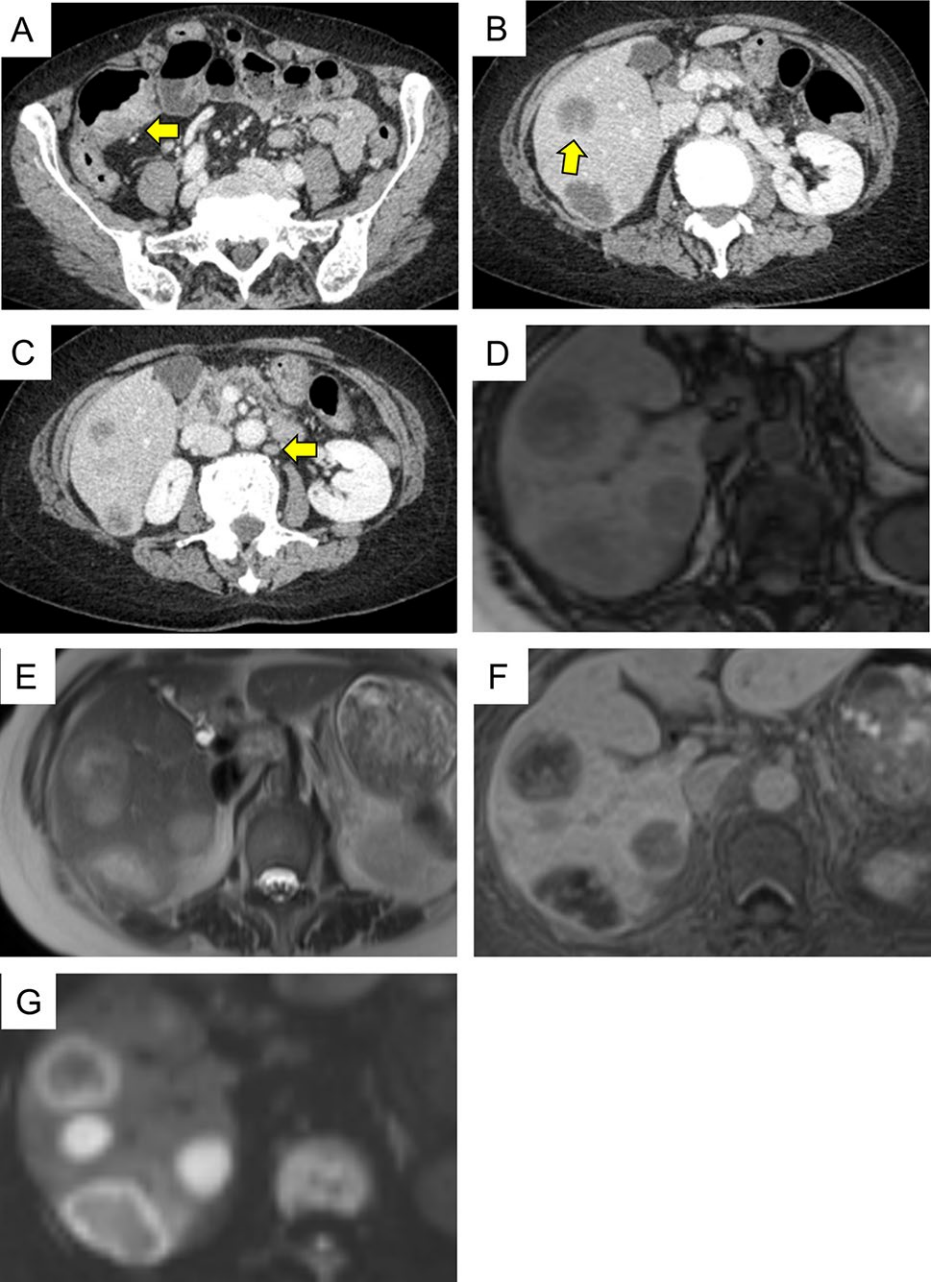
Abdominal imaging modalities. Contrast-enhanced computed tomography (CT) showed a mass with enhancement in the ileocecal region (A, *arrow*), and multiple nodular masses without enhancement in the liver (B) and para-aortic region (C, *arrow*). Penetrating vessels running through the tumor were detected in the liver (B, arrow). Contrast-enhanced magnetic resonance imaging (MRI) with gadolinium-ethoxybenzyl diethylenetriamine-penta-acetic acid (Gd-EOB-DTPA) showed that multiple tumors in the liver were lower contrast than the liver parenchyma in portal phase (D). T1-weighted images showed low signal in all lesions (E). The coarse lesion was centrally high signal on T2-weighted images (F) and low signal on DWI images (G)
